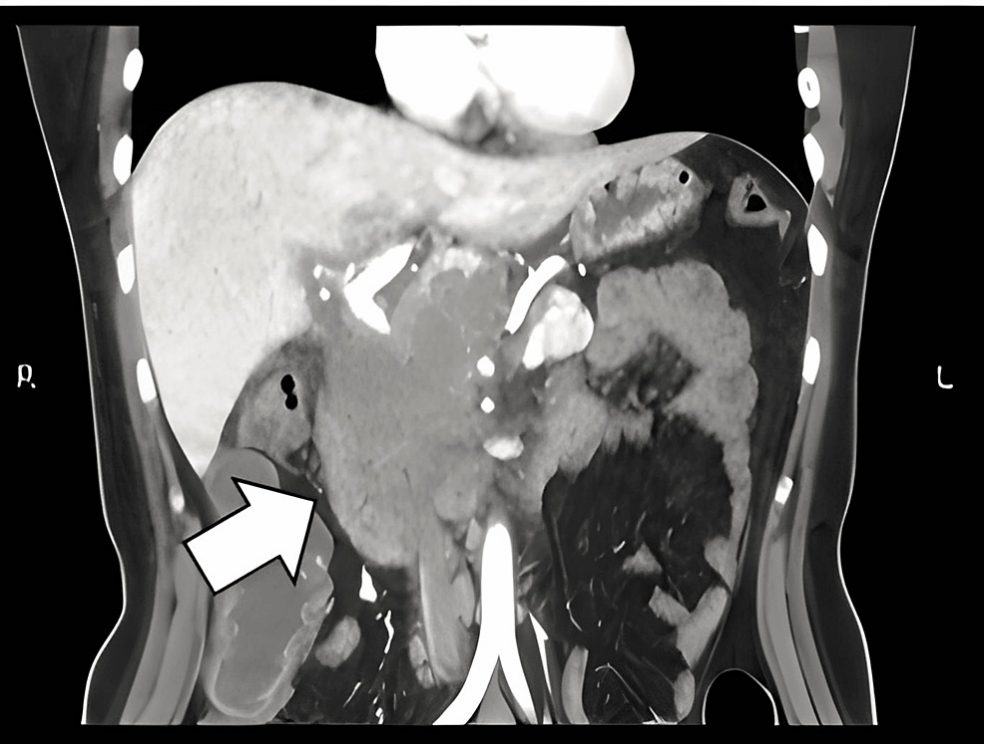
Multislice computed tomography (MSCT) of the pancreas showed adenopathic conglomerate with areas of central degeneration that reached up to 5 cm in a retroperitoneal, peripancreatic, cephalic, retroduodenal, and peri-aortic location. The largest volume was retroperitoneal which conditioned the anterior displacement of the pancreas.
